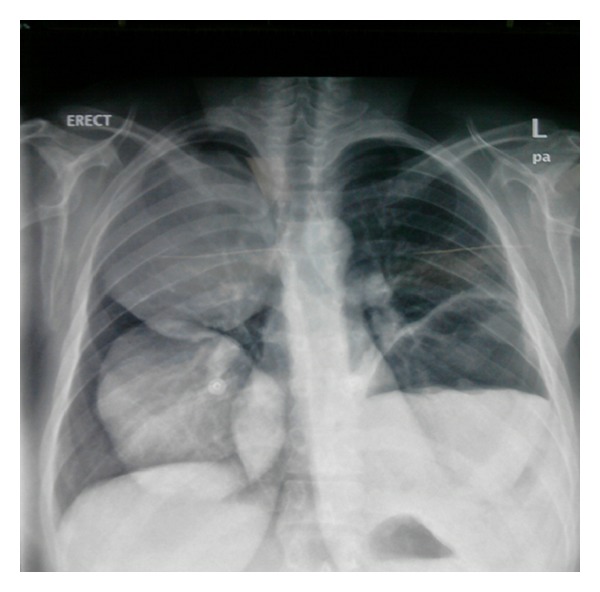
Chest X-ray revealed two fairly large unruptured hydatid cysts in the right lung and ruptured hydatid cyst in the left lung.
